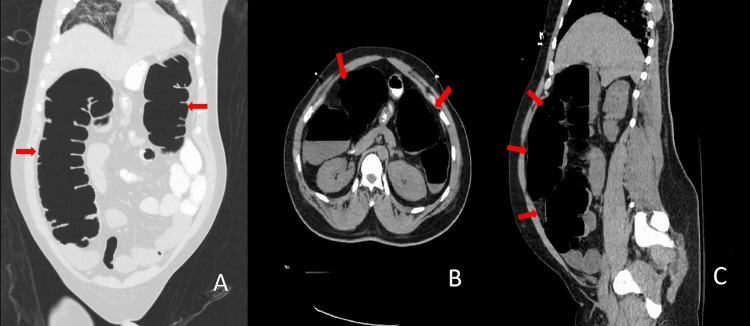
Abdominal CT scan: (A) coronal view; (B) axial view; (C) sagittal view Different views of abdominal CT scan demonstrate dilated loops of large bowel (red arrows).
